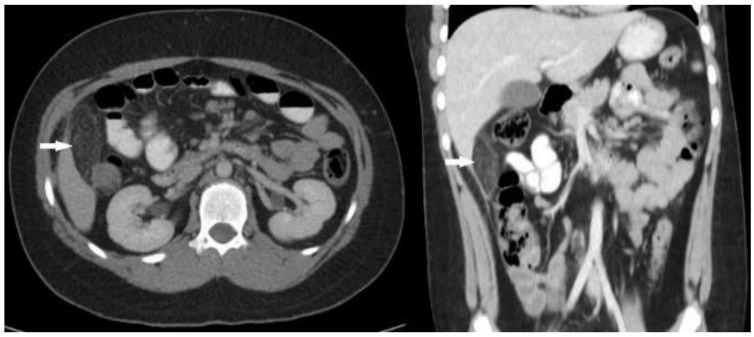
Axial (left) and coronal (right) CT images show an oval fat-attenuated mass (arrows) below the liver suggestive of OI.
